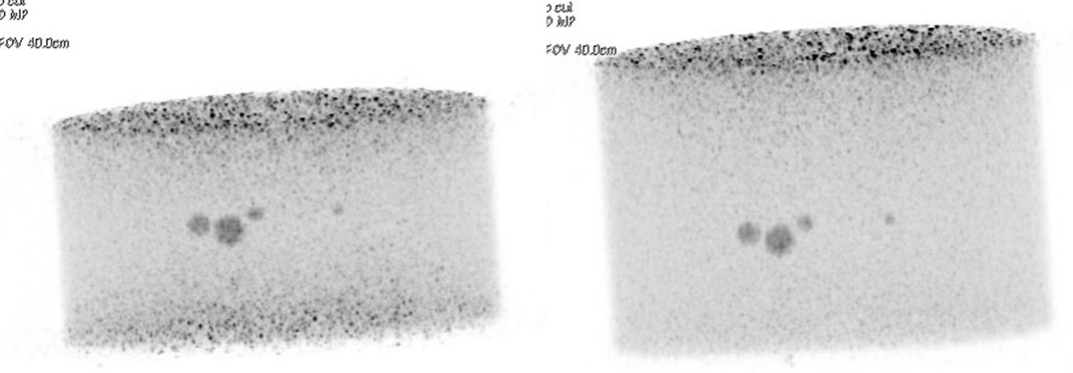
Three-dimensional maximum-intensity projection of equivalent frames (approx. 80 MBq) in the PET/CT (left) and PET/MR scanner (right)
